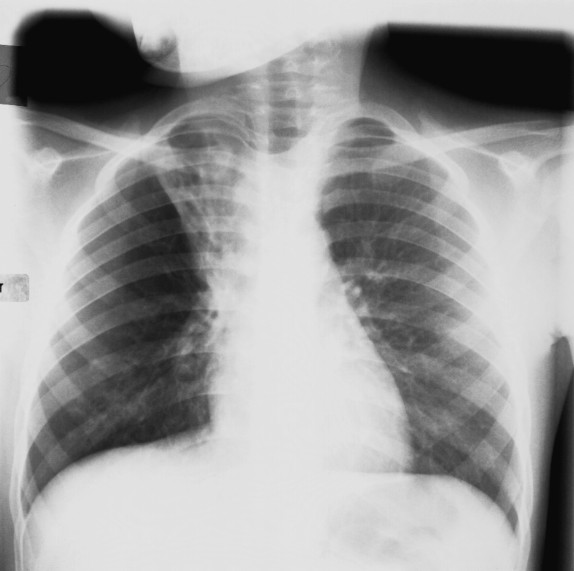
Chest x-ray of the child 4 months before referral. The CXR shows collapse and tram tracks of the right upper lobe and increased peribronchial and interstitial markings of the right lower lobe.
